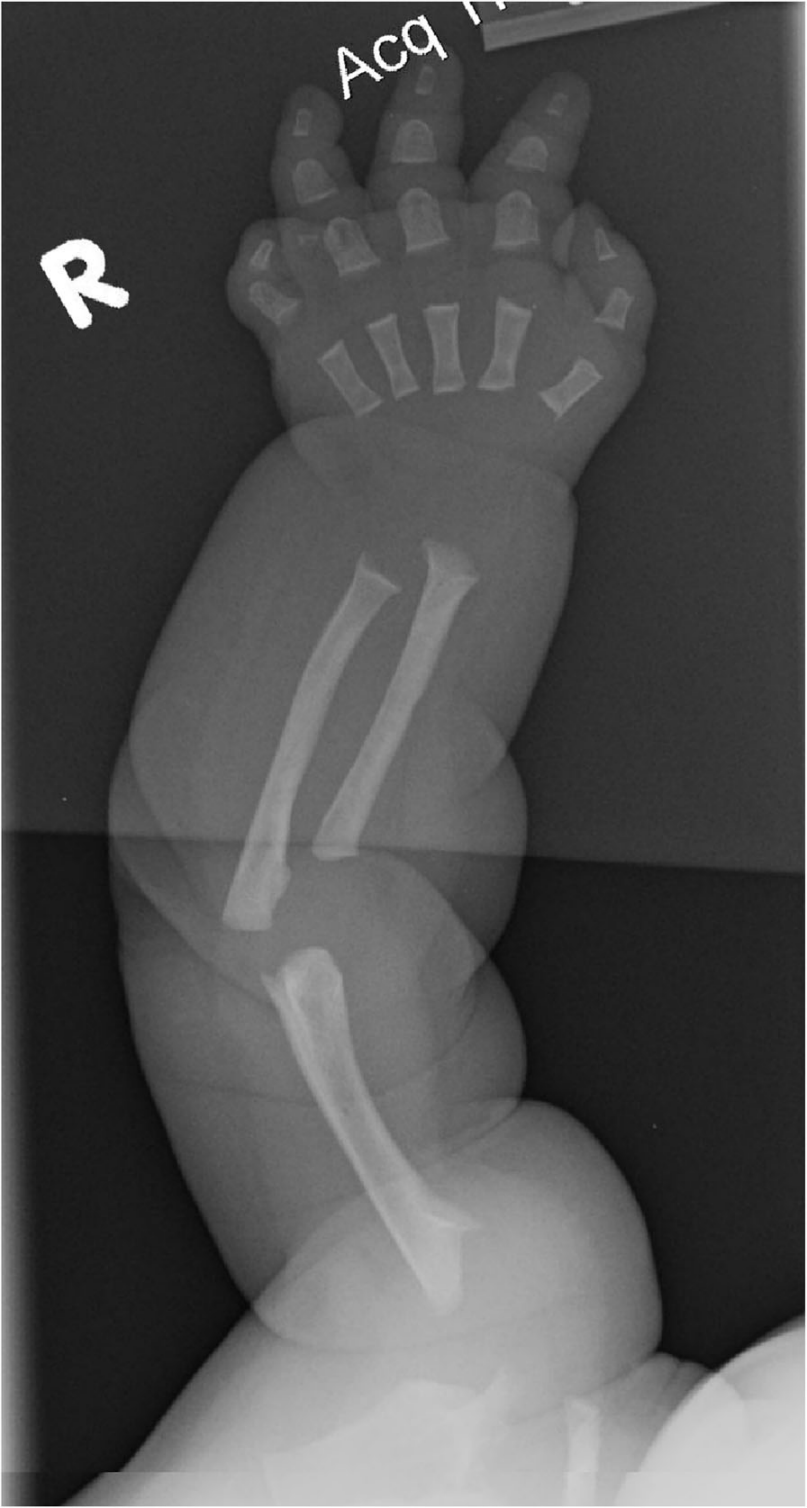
Arm radiograph in a newborn with achondroplasia. Although there are generalized metaphyseal abnormalities and shortening of all of the long bones, characteristics here are not as diagnostically helpful as those shown in Fig. 4
